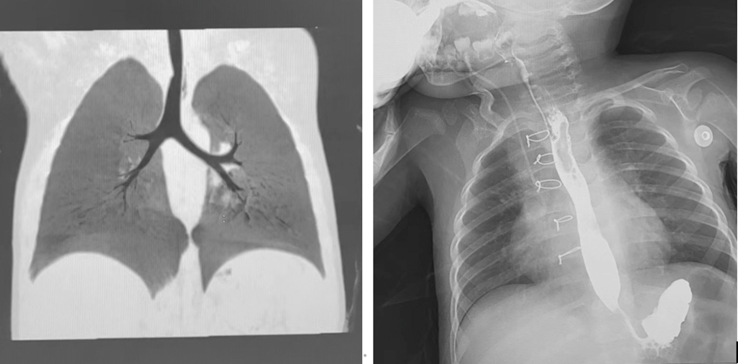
Follow-up via computed tomography (*left*) and gastrointestinal barium angiogram (*right*).
